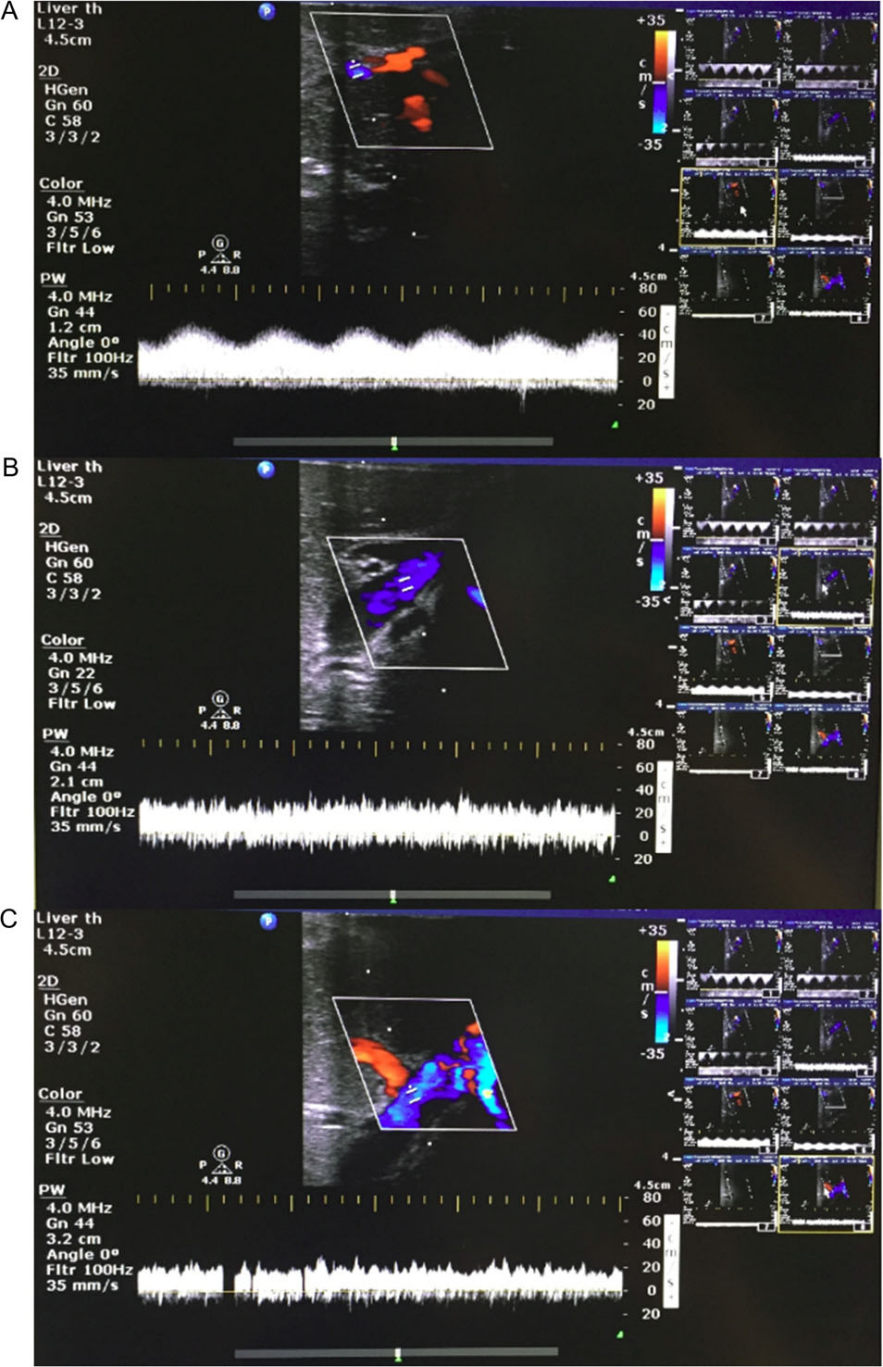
Representative Doppler ultrasound images of parenchyma from liver split with concurrent NMP-L. (A) Hepatic arteriolar waveform; (B) portal venous waveform; and (C) hepatic venous waveform.
